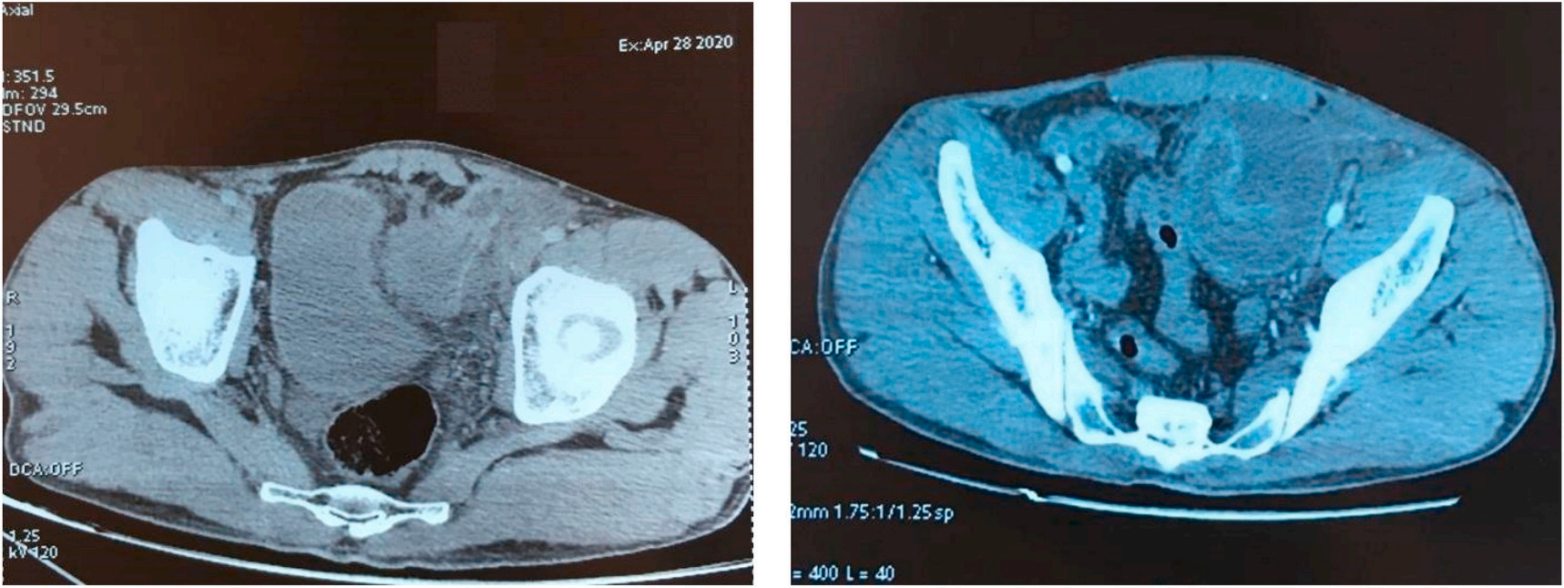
Abdomino pelvic CT scan objectivizing haemophilic invagination at the FIG level associated with a double hernia: internal supra bladder and left inguinal.
